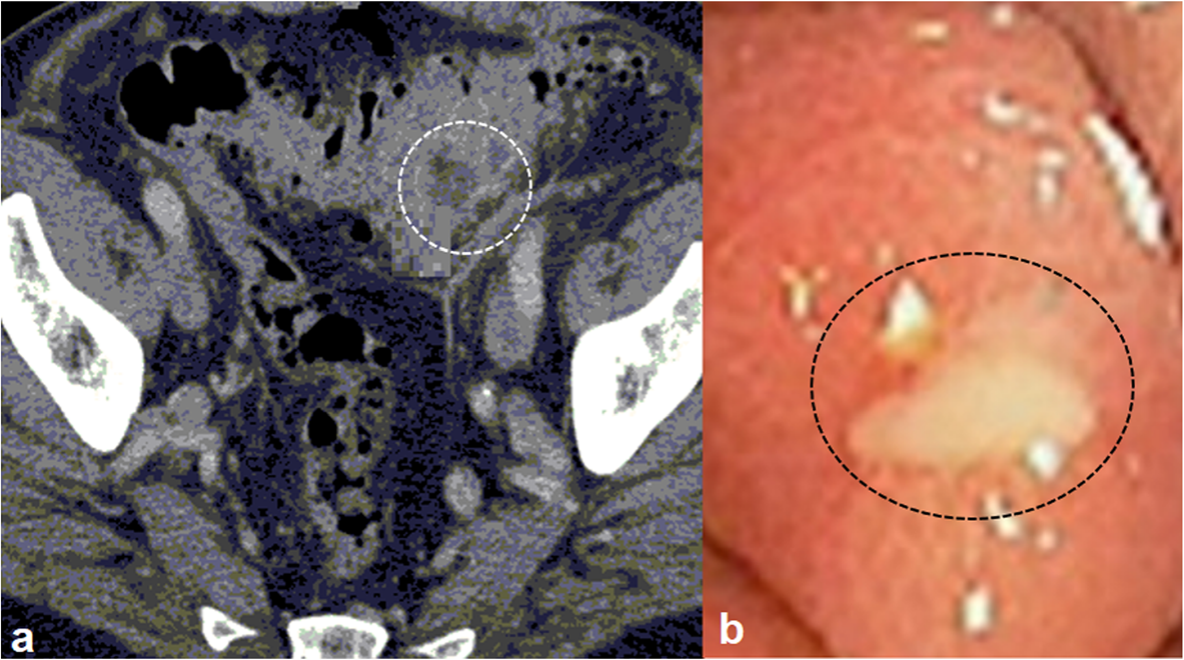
Lesion ulceration of male patients aged 51 years. a: Computed tomographic colonography, the white circle indicates lesion ulceration. b. Colonoscopy, the black circle indicates lesion ulceration
